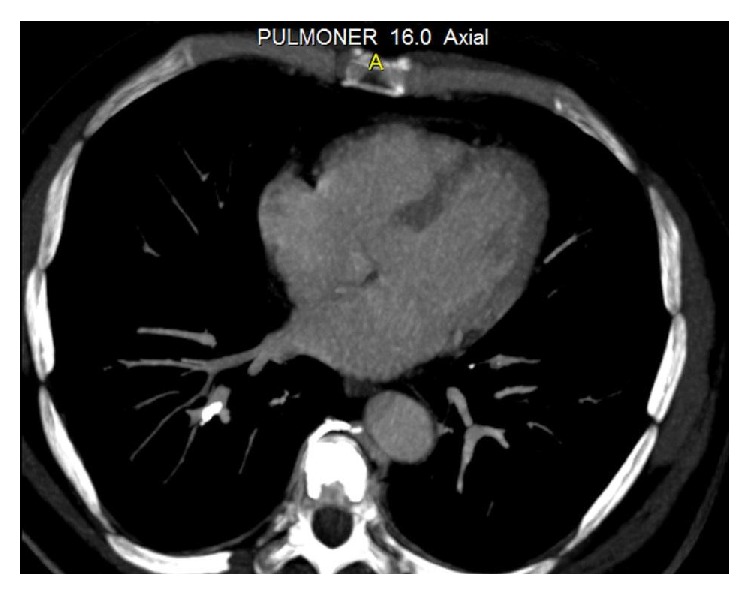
Cement leakage to the azygos vein was detected in thorax CT.
